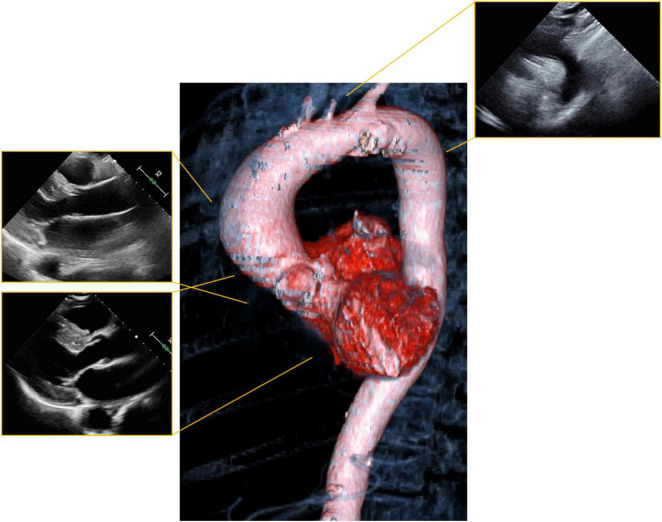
Visualization of the aorta with echocardiography. Standard parasternal and suprasternal notch views (periphery) leave a significant portion of the aorta unvisualized. Computer tomography (CT) or magnetic resonance imaging (MRI) can visualize the aorta in three dimensions (center) and allows for comprehensive evaluation of anatomy.
